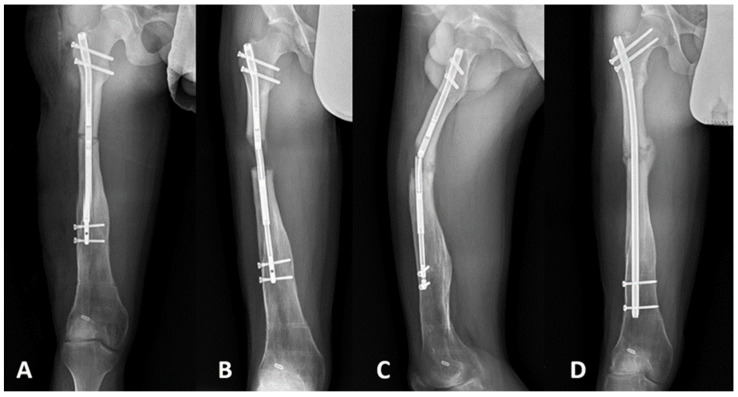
AP radiographs of a 15-year-old patient with CFD and 50 mm LLD qualified for femur lengthening with IMN Precise 2. (A) Postoperative AP radiograph. (B) AP radiograph after the end of distraction (before the regenerate consolidation). (C) Exposed broken IMN and regenerate. The patient did not follow the recommendations and went on a hike in mountains and started full weight bearing before the regenerate consolidation. (D) Fracture stabilization with the use of solid femoral nail—5 mm distraction was subsequently lost.
